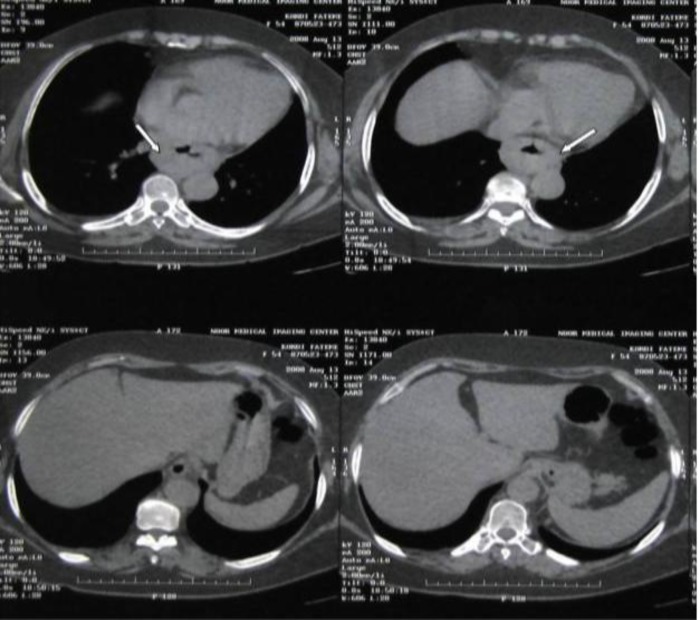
Computed tomography before surgery shows an esophageal mass (arrows
